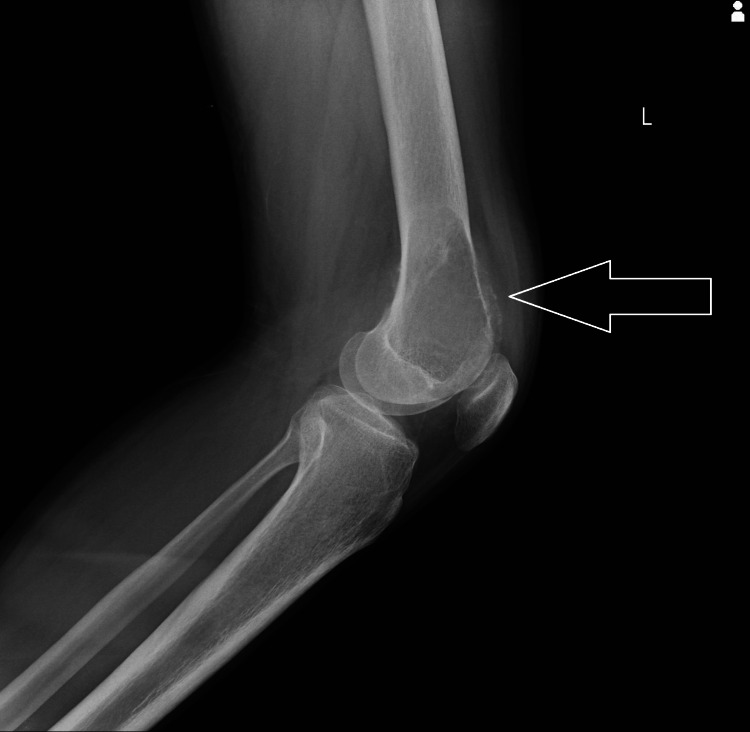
X-ray showing homogenous radio-opaque area with lytic appearance involving epiphysis of distal femur - lateral view
